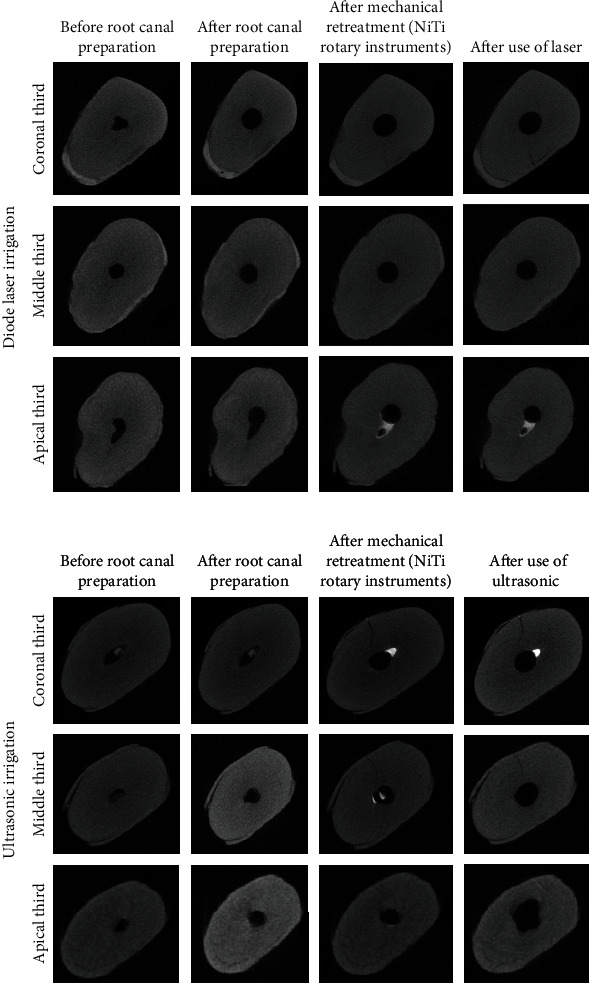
Micro-CT shows the crack formation and propagation in the same slice at four different timings using laser or ultrasonic irrigation.
